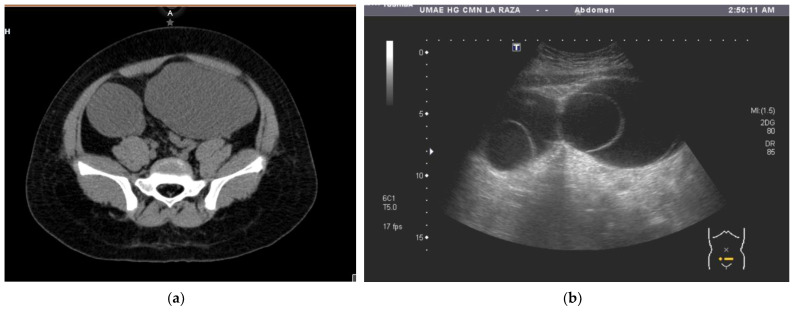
(a) Tomography image of the two pelvic tumors, A: Anterior; (b) ultrasound image of a pelvic tumor, T: Transductor.
